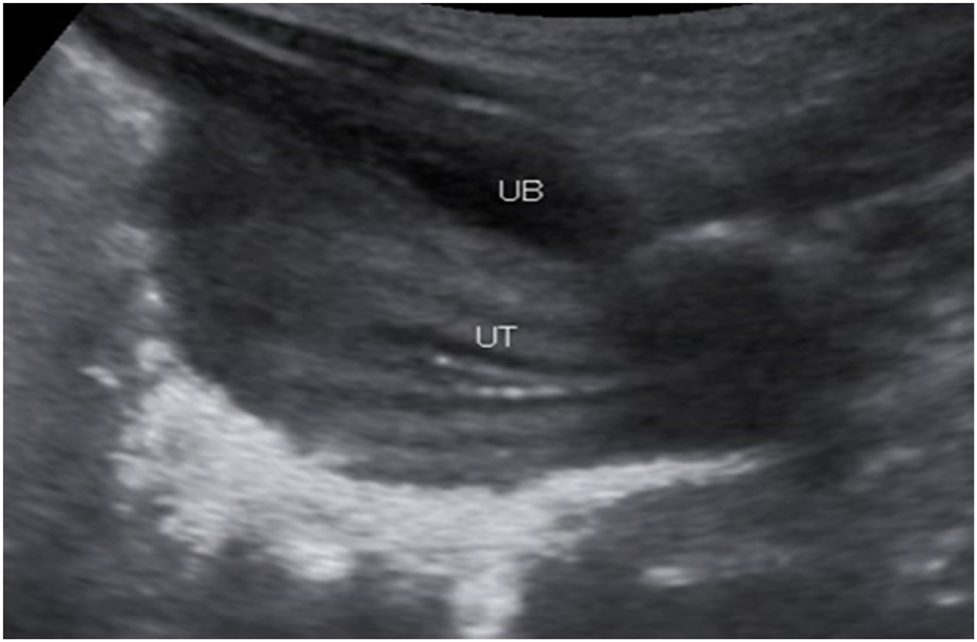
Neonatal abdominopelvic ultrasound showing empty uterus and vagina at the third post-operative day. UT, uterus; UB, urinary bladder.
